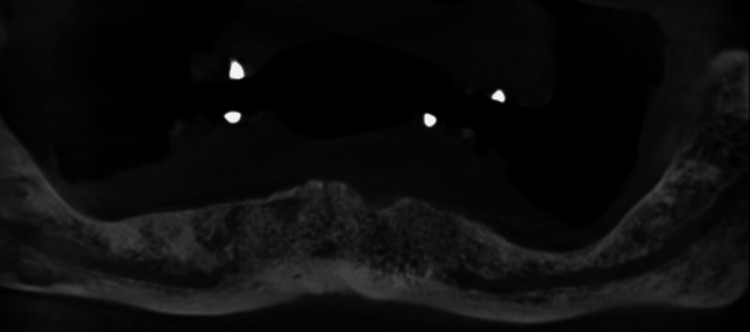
Panoramic reconstruction view obtained from preoperative CBCT. CBCT, cone beam computed tomography
